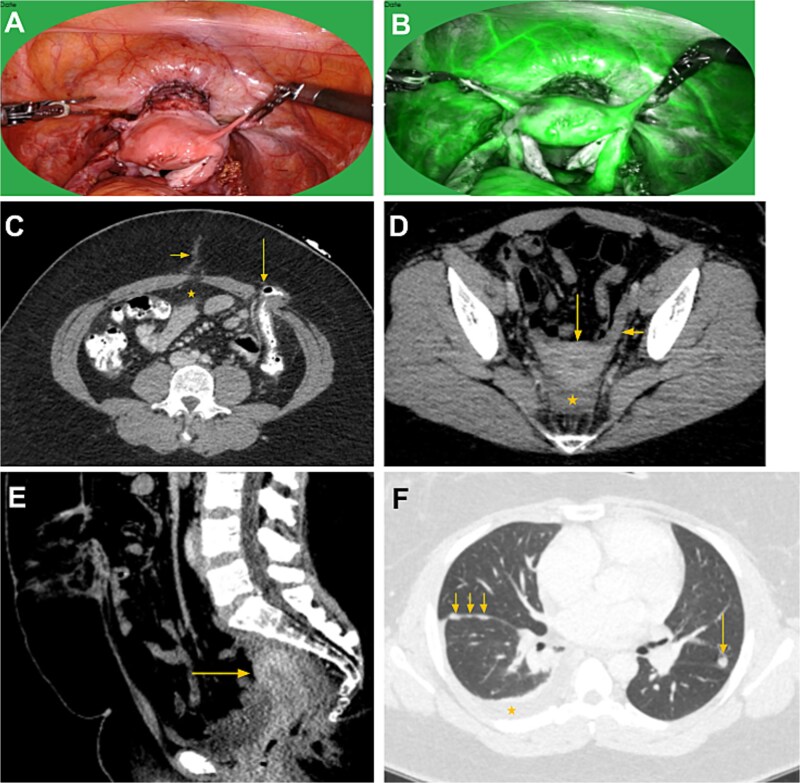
Intraoperative post reposition images (A and B) followed by post-reposition CT abdomen, pelvis, and chest. (C) Axial CT image at the level of the umbilicus shows minimal postsurgical stranding at the umbilical canal (short arrow). The previously transposed uterus is no longer present in the anterior midline abdomen (*). A left abdominal wall end colostomy is noted (long arrow), status post abdominoperineal resection. (D, E) Axial and sagittal pelvic images demonstrate the uterus (long arrows) repositioned in its anatomical location. The left utero-ovarian ligament is visualized (short arrow in D). A small amount of free fluid is seen in the abdominoperineal resection bed (* in E). Ovaries (not shown) have also been repositioned to their anatomical locations. (F) CT chest performed 2 months post-reposition demonstrates metastatic disease to the lungs and right pleura, including a right pleural effusion (*), right fissural nodularity (short arrows), and a left lung nodule (long arrow).
